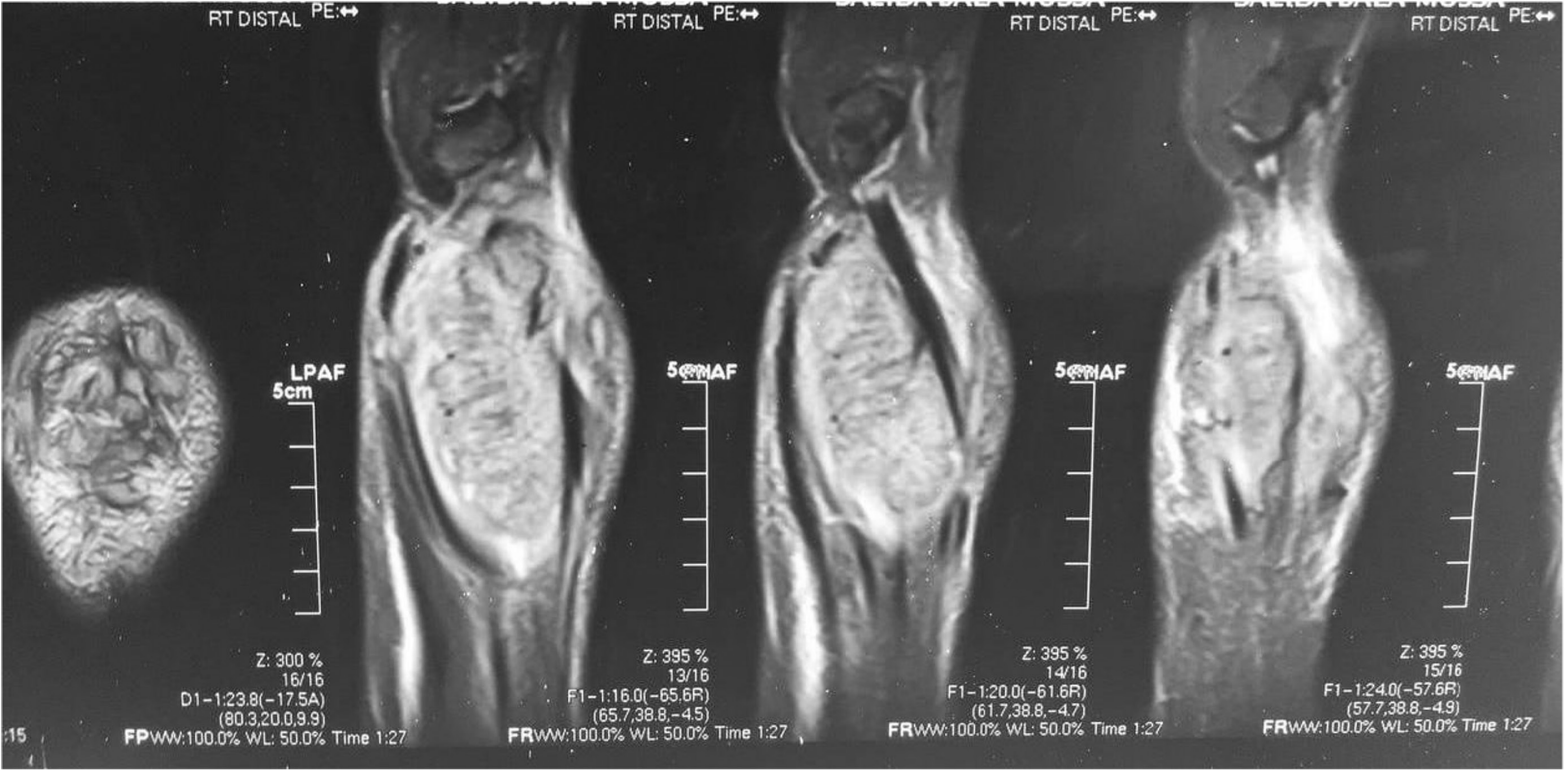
Preoperative MRI sagittal view of Rt forearm shows right distal forearm swelling (enhancement) incasing distal neurovascular bundle, pretumor edema, and no skip lesions.
